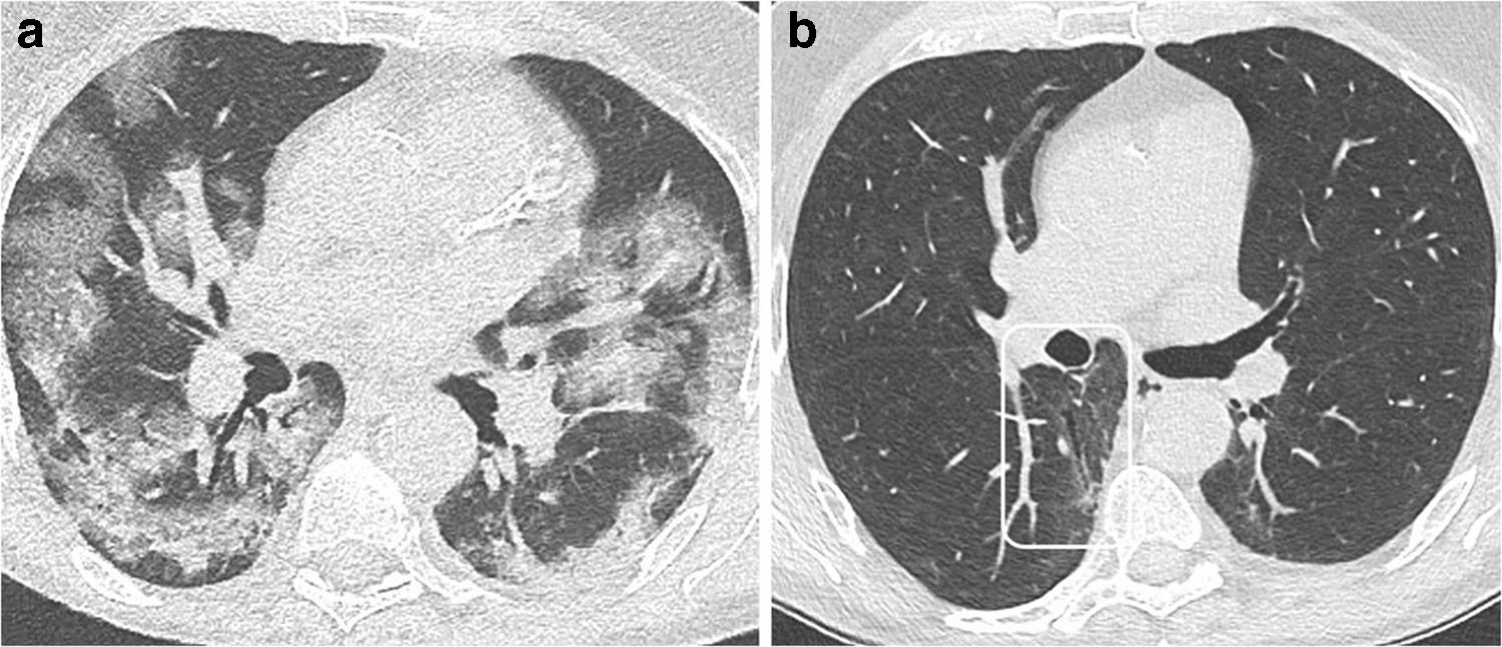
CT features at baseline, and 1-year follow-up, in a 63-year-old man who developed severe COVID-19 pneumonia (intubation, 9 days in ICU). a Baseline CT. b 1-year CT follow-up. Ground glass opacities (GGOs) have largely resolved. Mild bronchial dilatation (rectangle) is demonstrated within the residual GGO area of the right lower lobe. Pulmonary function tests are normal, with a DLCO value of 90%
